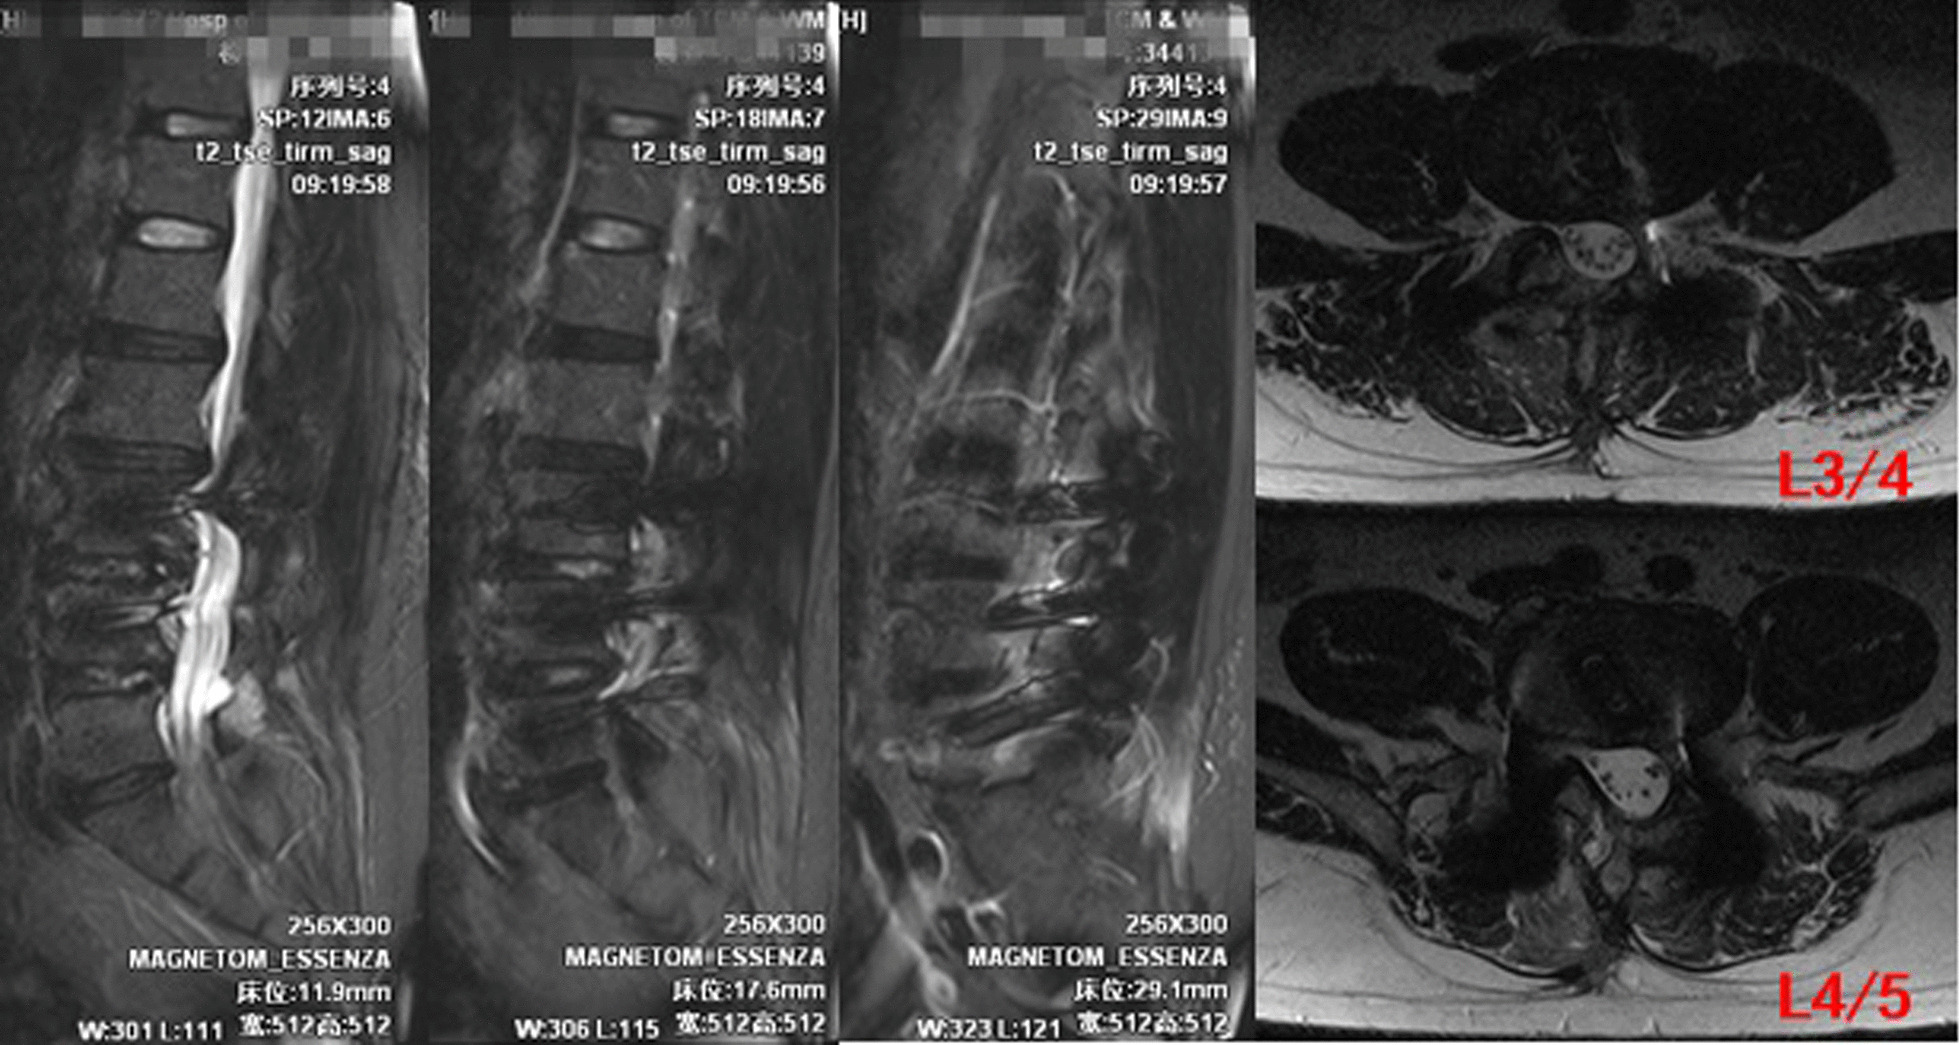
MRI was re-examined more than 3 months after the operation
